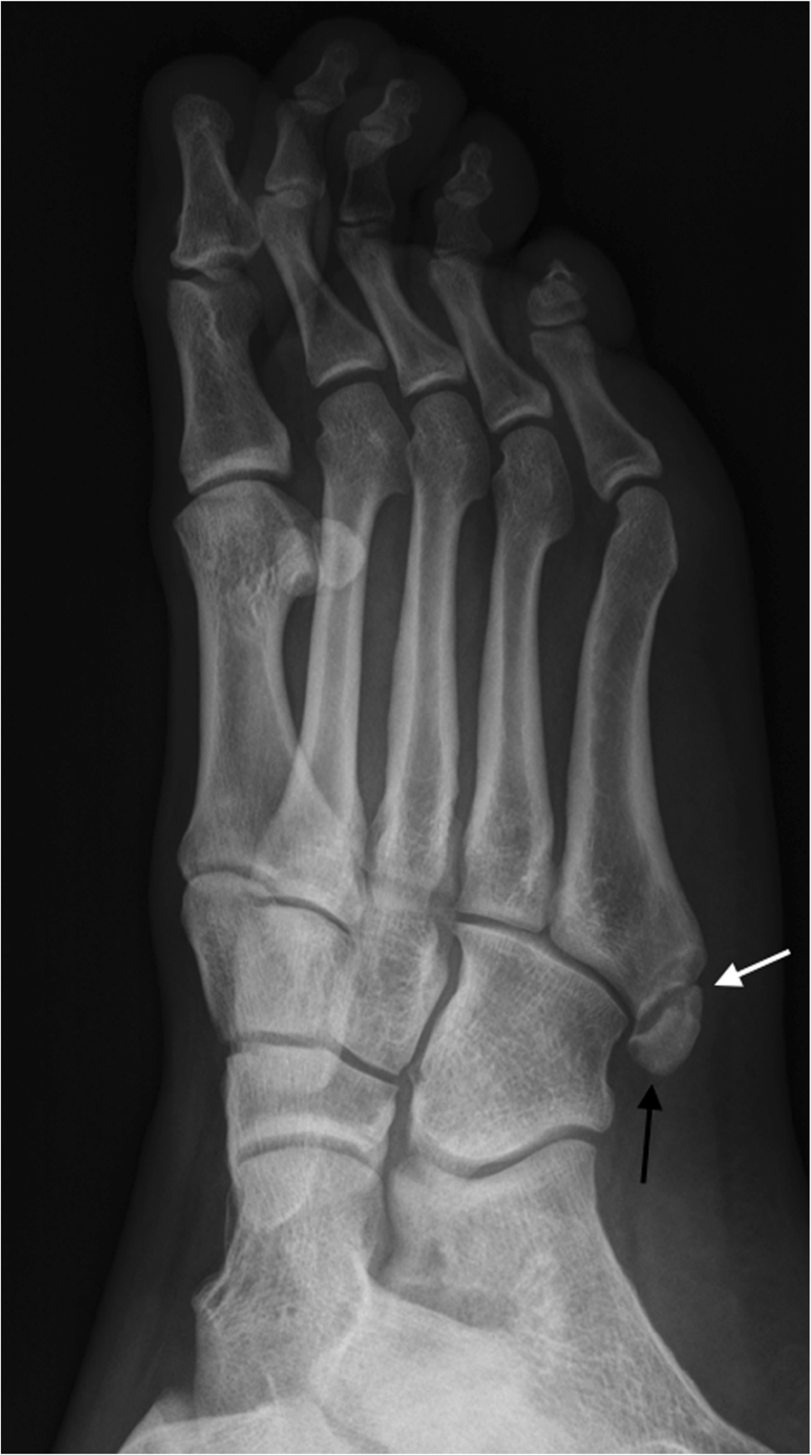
Differential of os vesalianum. Oblique radiograph. Old non-united fracture fragment of the apophysis of the fifth metatarsal (black arrow). The fracture line is visible (white arrow). This finding, in some occasions, can resemble an os vesalianum
